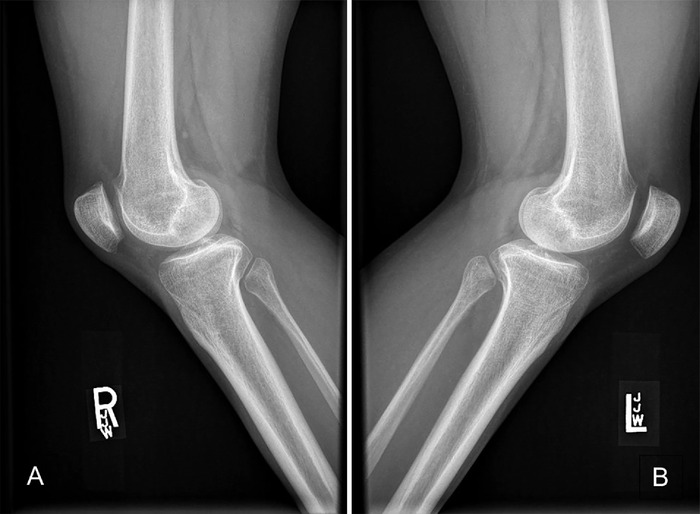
Preoperative lateral radiographic images show (A) right knee with a Caton-Deschamps index of 1.27 and (B) left knee with a Caton-Deschamps index of 1.20.
